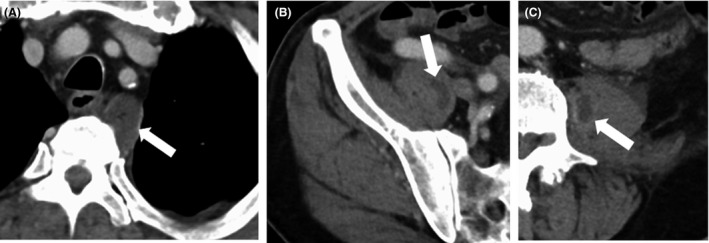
Computed tomography with contrast on post‐admission day 6 in a 65‐year‐old man admitted with meningeal irritation. Abscesses are seen in the mediastinum (A) (arrows) and bilateral psoas muscles (B) (arrows).
